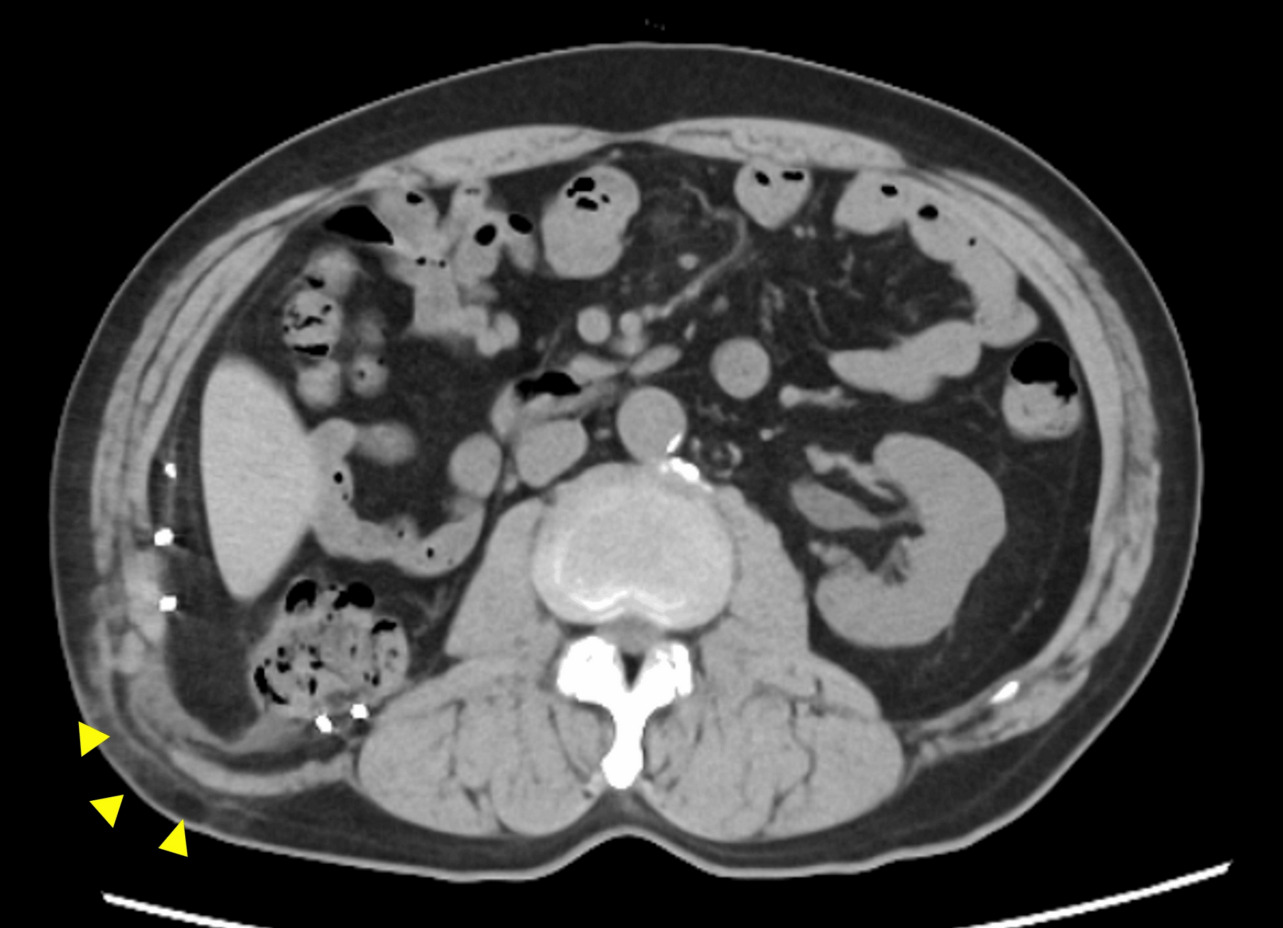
Computed tomography image obtained 36 months after the surgery A slight mesh bulge without herniation of visceral organs is observed (yellow arrowheads).
